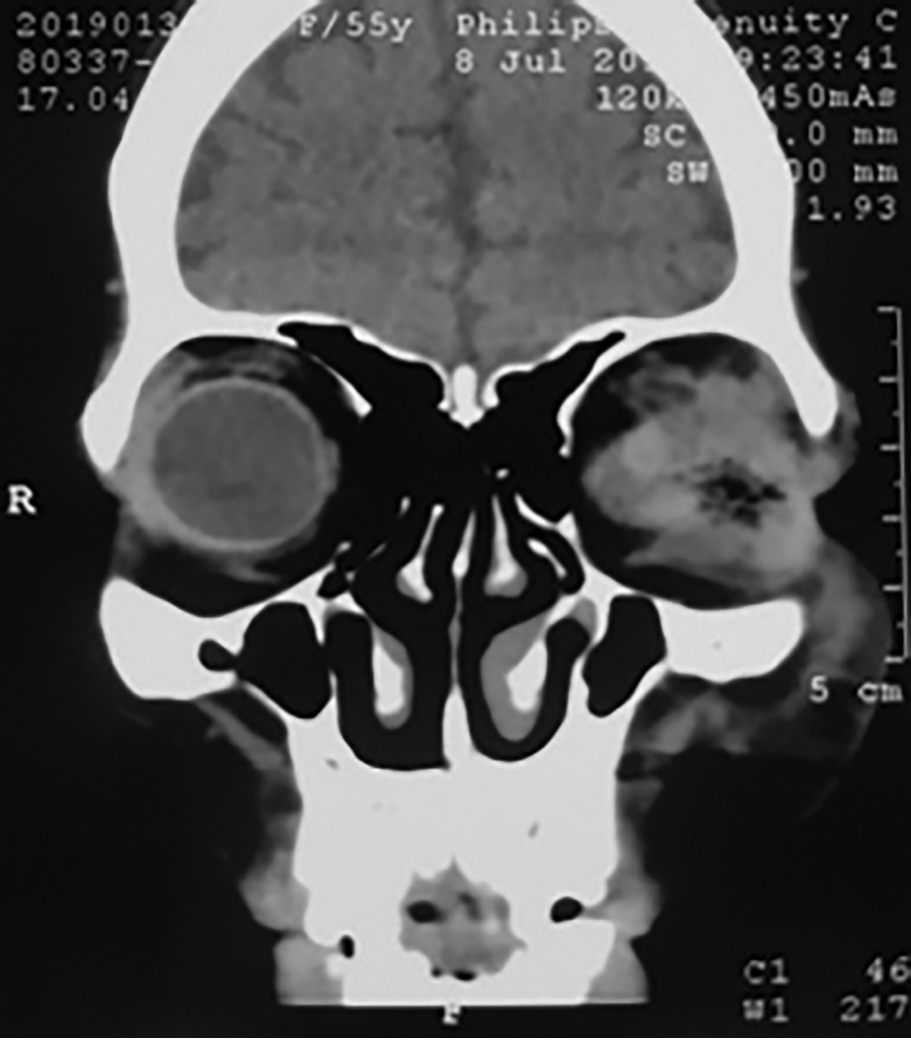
CT scan of the orbit and head CT: Computed tomography
